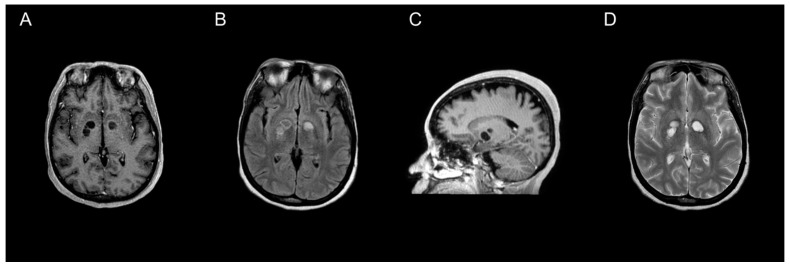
MRI characterization of cryptococcomas. MRI of the brain showing a nonenhancing cryptococcoma (axial plane T1-weighted post contrast (A), axial plane T2-FLAIR (B), post-contrast parasagittal (C), and axial plane T2-weighted (D)).
